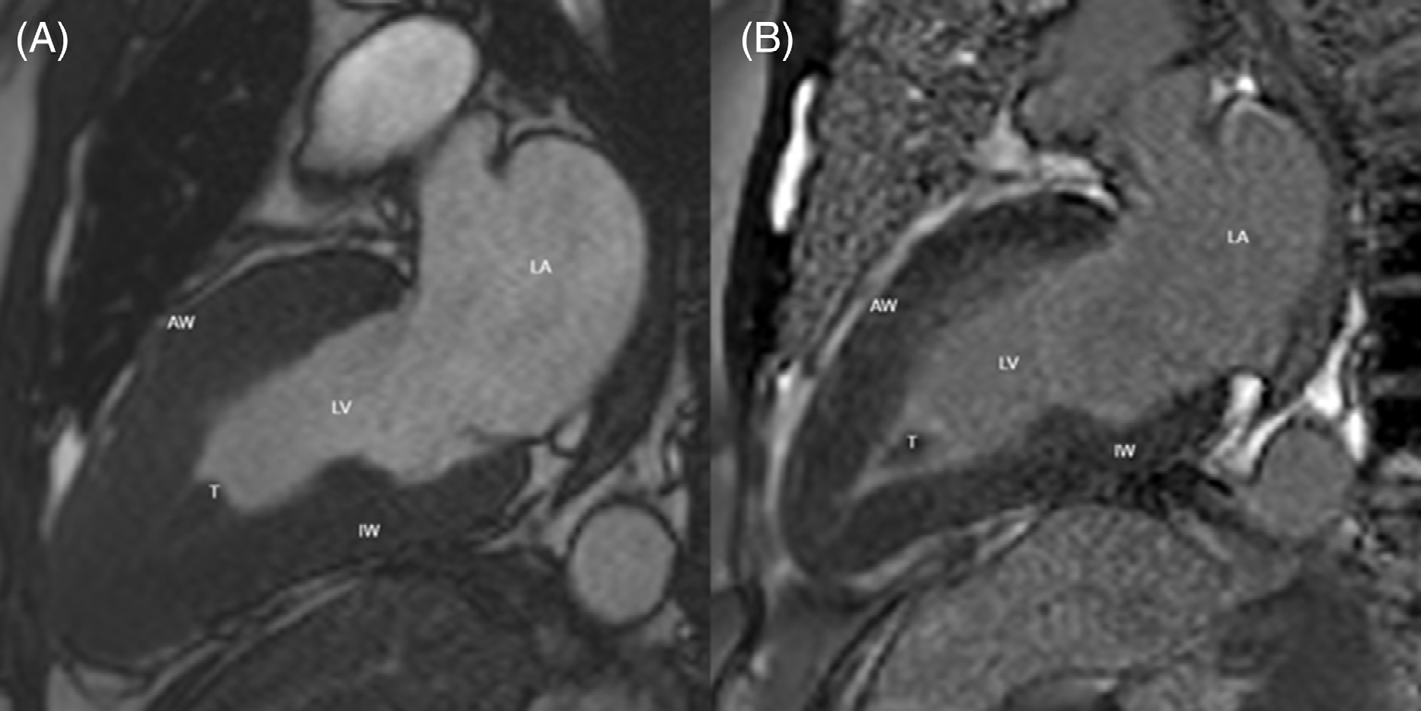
Long axis 2 chamber view on cardiac magnetic resonance imaging (cMRI) pre gadolinium cine image (a) and post gadolinium (b). IW, inferoseptal wall; AW, anterolateral wall; T, thrombus; LV, left ventricle; LA, left atrium; RV, right ventricle; RA, right atrium
